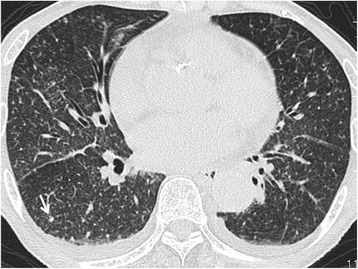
Chest CT on admission showed diffuse micronodules at random pattern in both lung field and a small nodule in the right S6 (white arrow)
